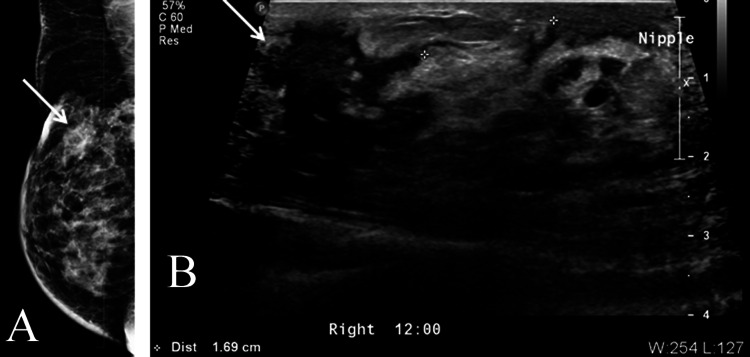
A 55-year-old male with right breast mediolateral oblique mammograms showing prominent gynecomastia concomitant with very suspicious mass (breast imaging-reporting and data system [BI-RADS] 5) at the upper outer quadrant (arrowed) with skin retraction confirmed in ultrasound, invasive ductal carcinoma, hormone receptor-positive and human epidermal growth factor receptor 2 (HER2) negative. (A) Right breast mammogram mediolateral oblique view. (B) Corresponding ultrasound of the right breast.
